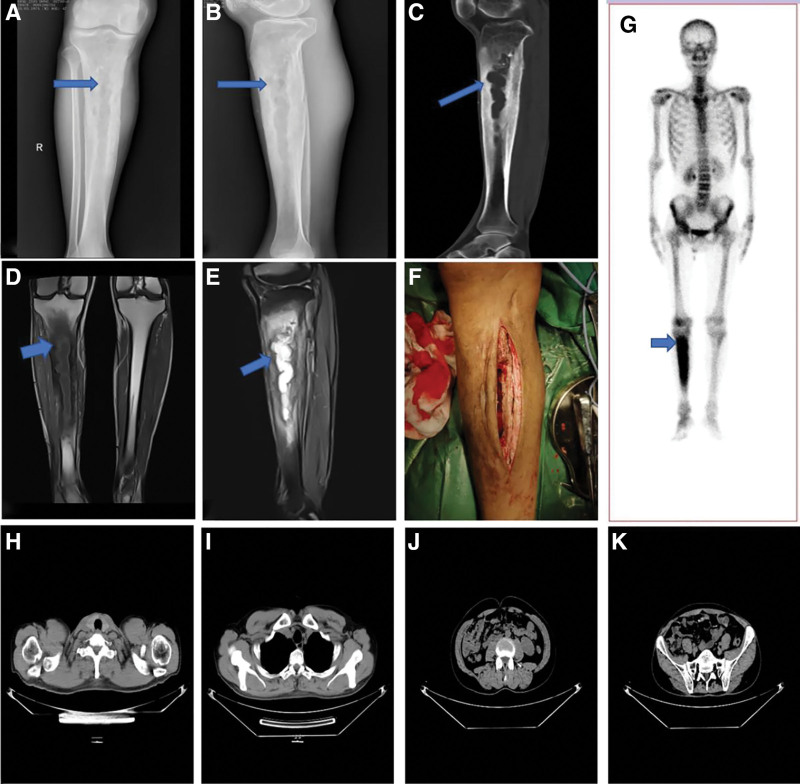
(A and B) Anteroposterior and lateral radiographic views of the proximal tibia show dead bone, an increased marrow cavity, cortical bone thickening, no periosteal reaction, and no soft tissue, representing an aggressive lesion (arrow). (C) A computed tomography (CT) scan shows an enlarged medullary cavity of the middle and upper part of the tibial shaft. Also, the normal bone trabecular structure disappeared, and the bone cortex is obviously thickened and hardened (arrow). (D) Spin echo T1-weighted magnetic resonance imaging (MRI; coronal view) of both legs (for comparison) for chronic osteomyelitis shows cortical thickening, bone-marrow edema, and a sequestrum (arrow) on the right tibia (arrow). (E) A T2-weighted MRI image shows hyperintensity and short tau inversion recovery sequences (arrow). (F) Intraoperative imaging depicts debridement, dead bone removal, and fenestration and drainage of the medullary cavity. (G) An ECT scan shows apparent, concentrated radioactivity in the upper tibia (arrow). (H–K) CT scans of the cervical vertebra (H), thoracic vertebra (I), lumbar vertebra (J), and pelvis (K) show no bone destruction and typical plasma cell tumor lesions. ECT = emission computed tomography.
